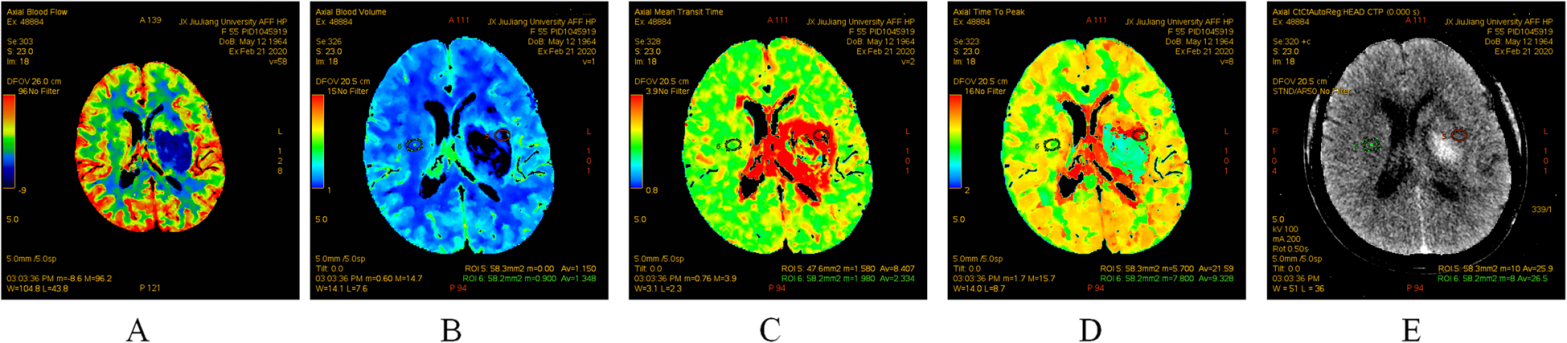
Maps of CT and CTP in an ICH patient example. a Cerebral blood flow (CBF). b Cerebral blood volume (CBV). b Mean transition time (MTT). d Time to peak (TTP). e CT brain scanning
